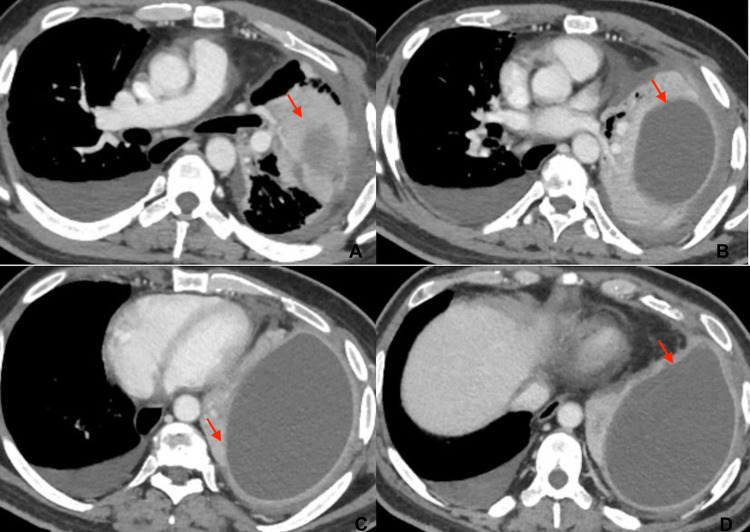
A-D. Axial sections of chest CT scan showing a left chronic thick-walled Stage III empyema.
